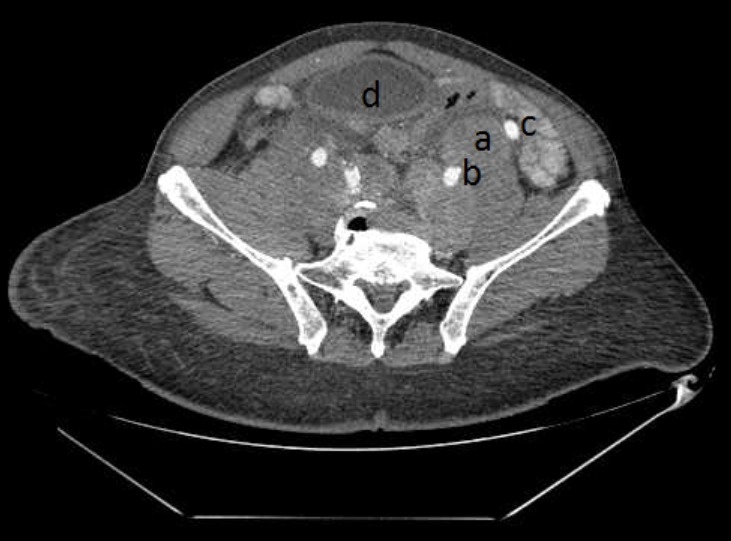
Contrast enhanced pelvic CT scan showing (a) a huge heterogenous dense structure (prostate gland) indenting the posterior bladder wall
